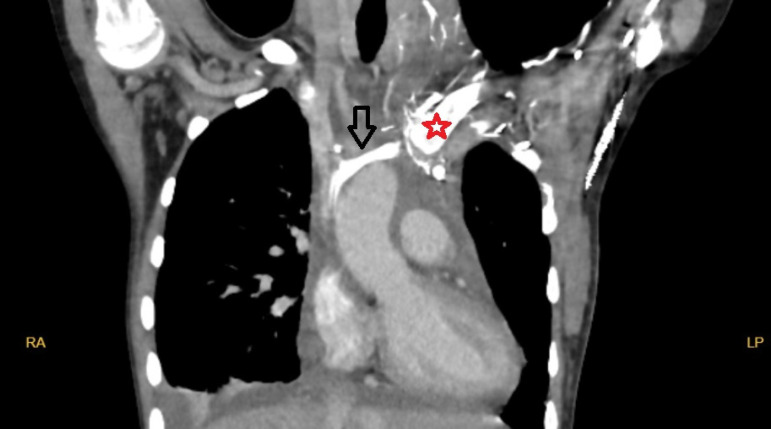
Computed tomography angiographic image of the persistent left
